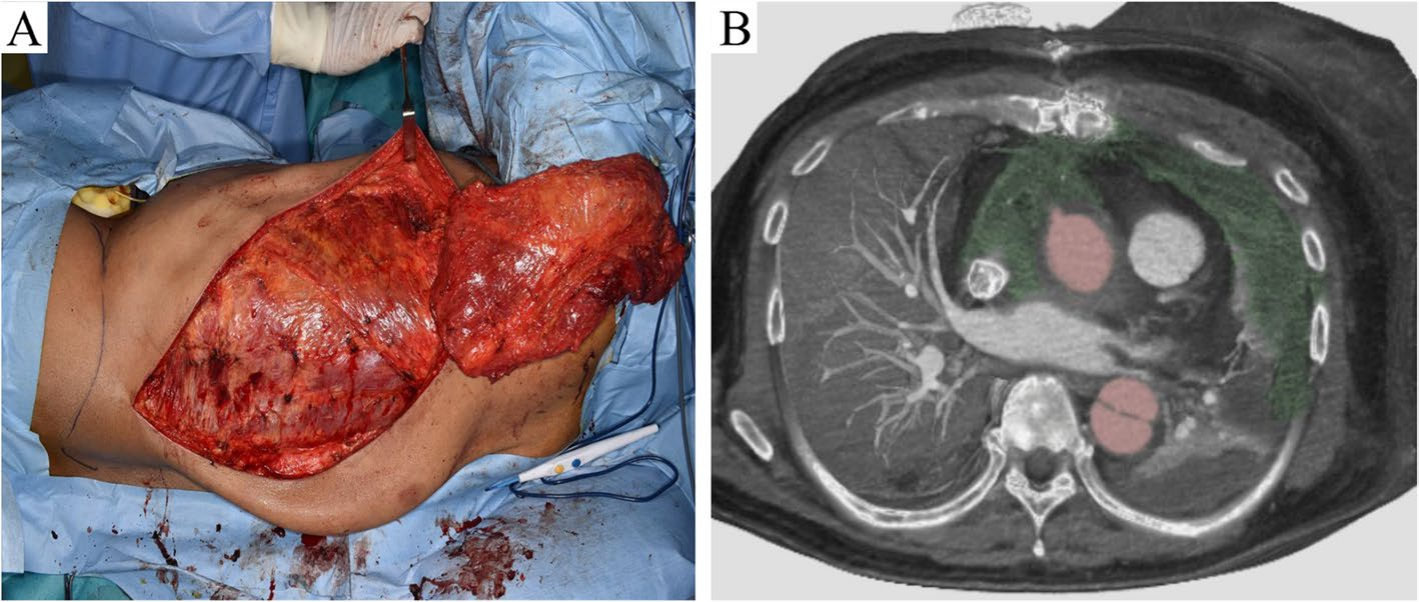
Latissimus dorsi flap grafting. A Latissimus dorsi flap was prepared 8 days after operation. B Postoperative CT findings after implantation of the latissimus dorsi flap (green area)
